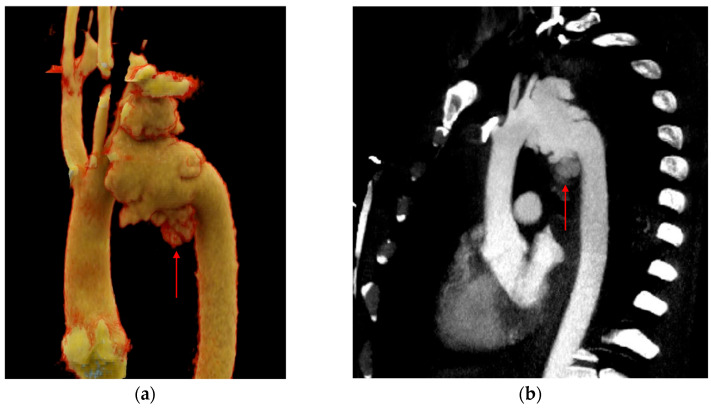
Contrast extravasation. (a) 3D volume-rendered and (b) sagittal CTA images of the chest demonstrate aneurysmal dilatation at the distal arch with extravasation of contrast beyond the aortic contour (red arrows).
